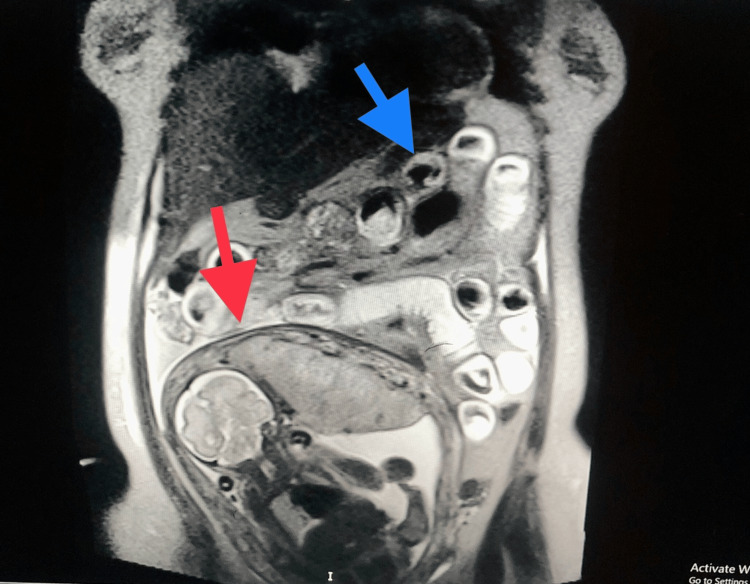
MRI scan The MRI scan image confirms small bowel obstruction. Dilated small bowel loops were seen in the upper quadrants (blue arrow). The fetus is seen in utero (red arrow).
